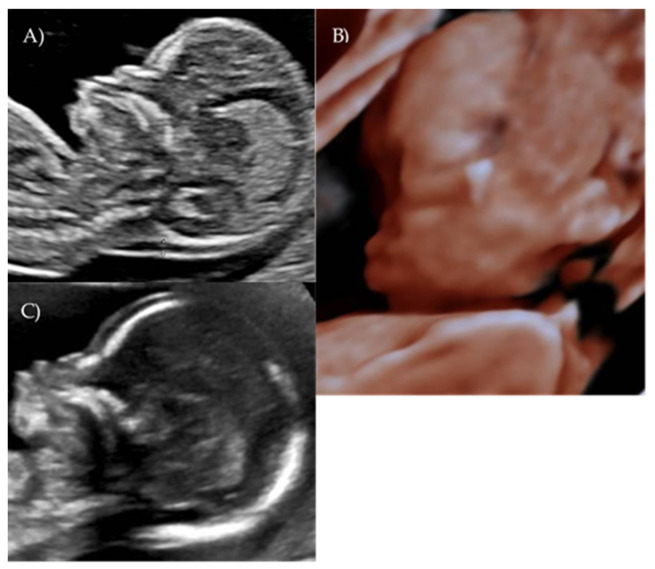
Case 2.* (A) First-trimester 2D ultrasound fetal profile. (B) Flat fetal profile, 3D reconstruction, at 17 + 4 w. (C) Flat fetal profile, 17 + 4 w.
